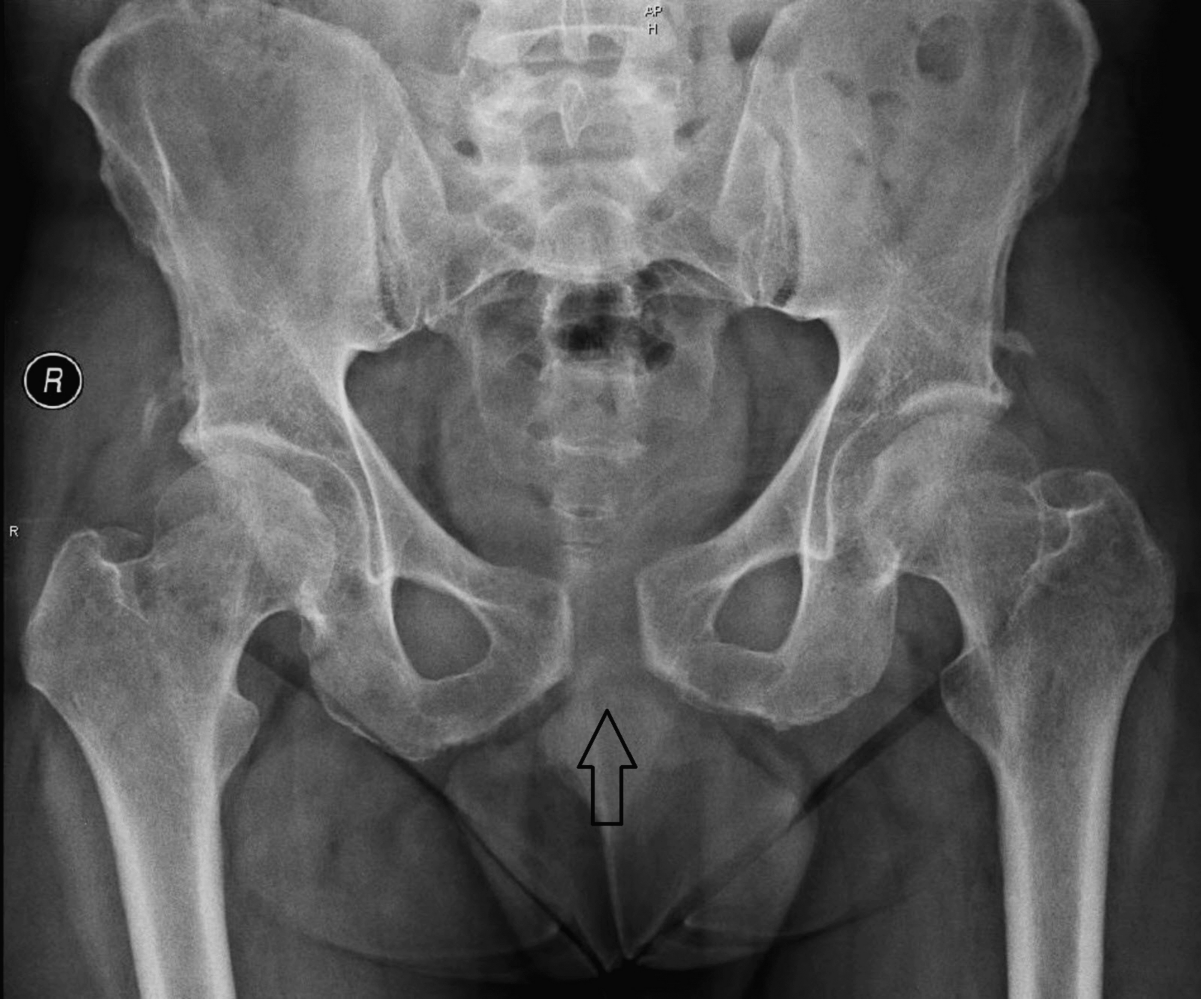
Anteroposterior radiograph of pelvis eight months after injury showing no further loss of reduction.
